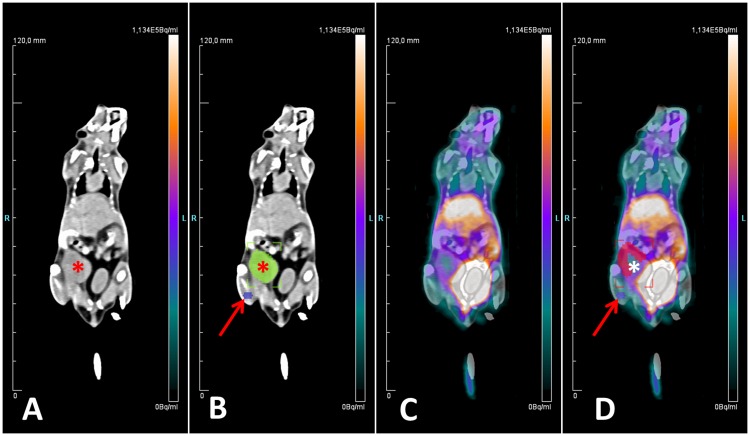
VOI selection. Images in coronal reconstruction. A: unenhanced CT (tumor indicated by asterisk); B: unenhanced CT with tumor (green, asterisk) and muscle (violet, arrow) VOIs; C: fused PET and CT data sets; D: fused PET and CT data sets with tumor (pink, asterisk) and muscle (violet, arrow) VOIs. Tumor and muscle VOIs selected on the unenhanced CT (B) were superimposed on the PET data sets (D) to allow for a quantification of the PET signal. Note that tumor regions with significant signal spillover from the urinary bladder were excluded from the quantitative analysis (D).
